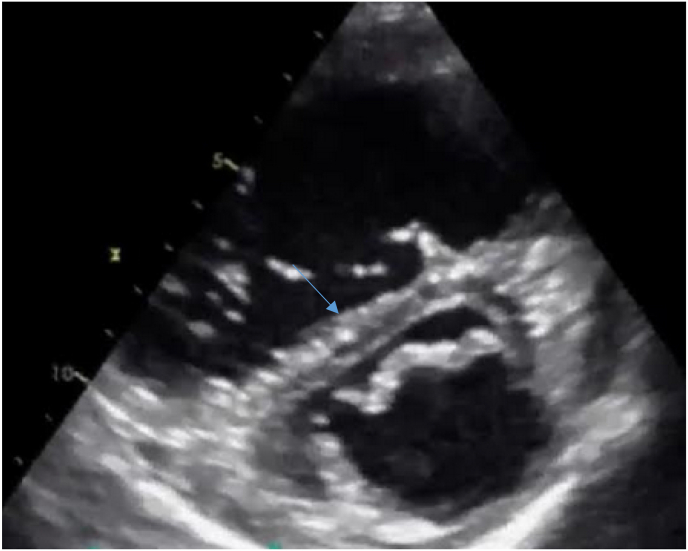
Short axis parasternal section showing dilatation of the right ventricle associated with flattening of the interventricular septum.
